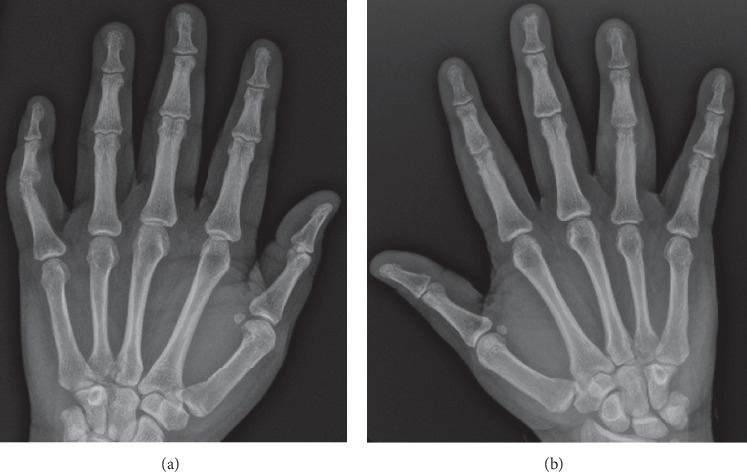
(a) X-ray of the left hand with erosive changes at the 5^th^ PIP joint. (b) X-ray of the right hand with erosive changes at the 2^nd^ and 4^th^ PIP joints.
